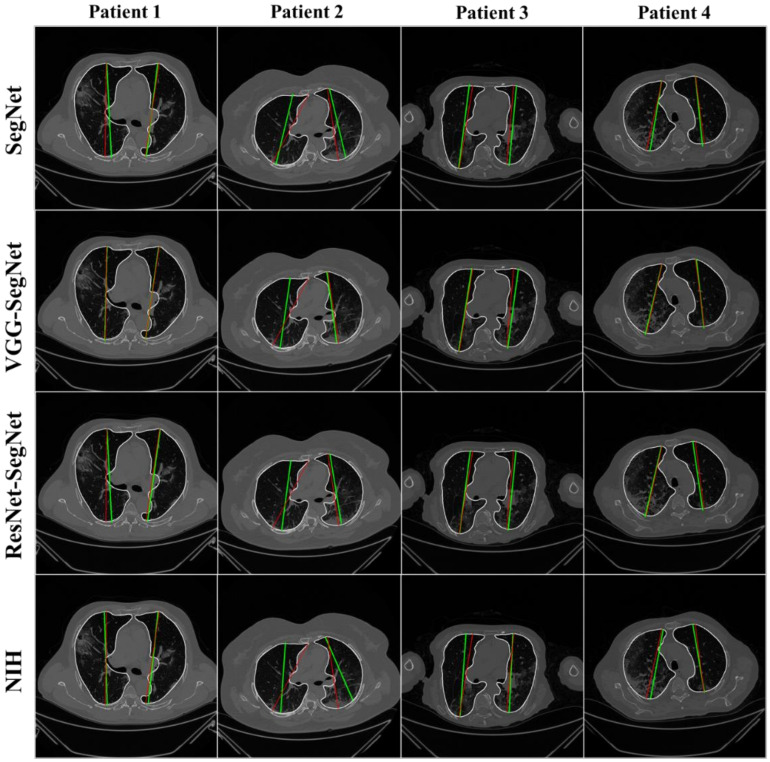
Display of the AI-long axis (green) and GT-long axis (red) showing the reach of the top apical zone and bottom basal zone of the left and right lungs. Rows are the four models, and columns are sample four patients COVID-19 CT scans. White is the GT delineated borders of the lungs by the tracer using ImgTracer(TM).
